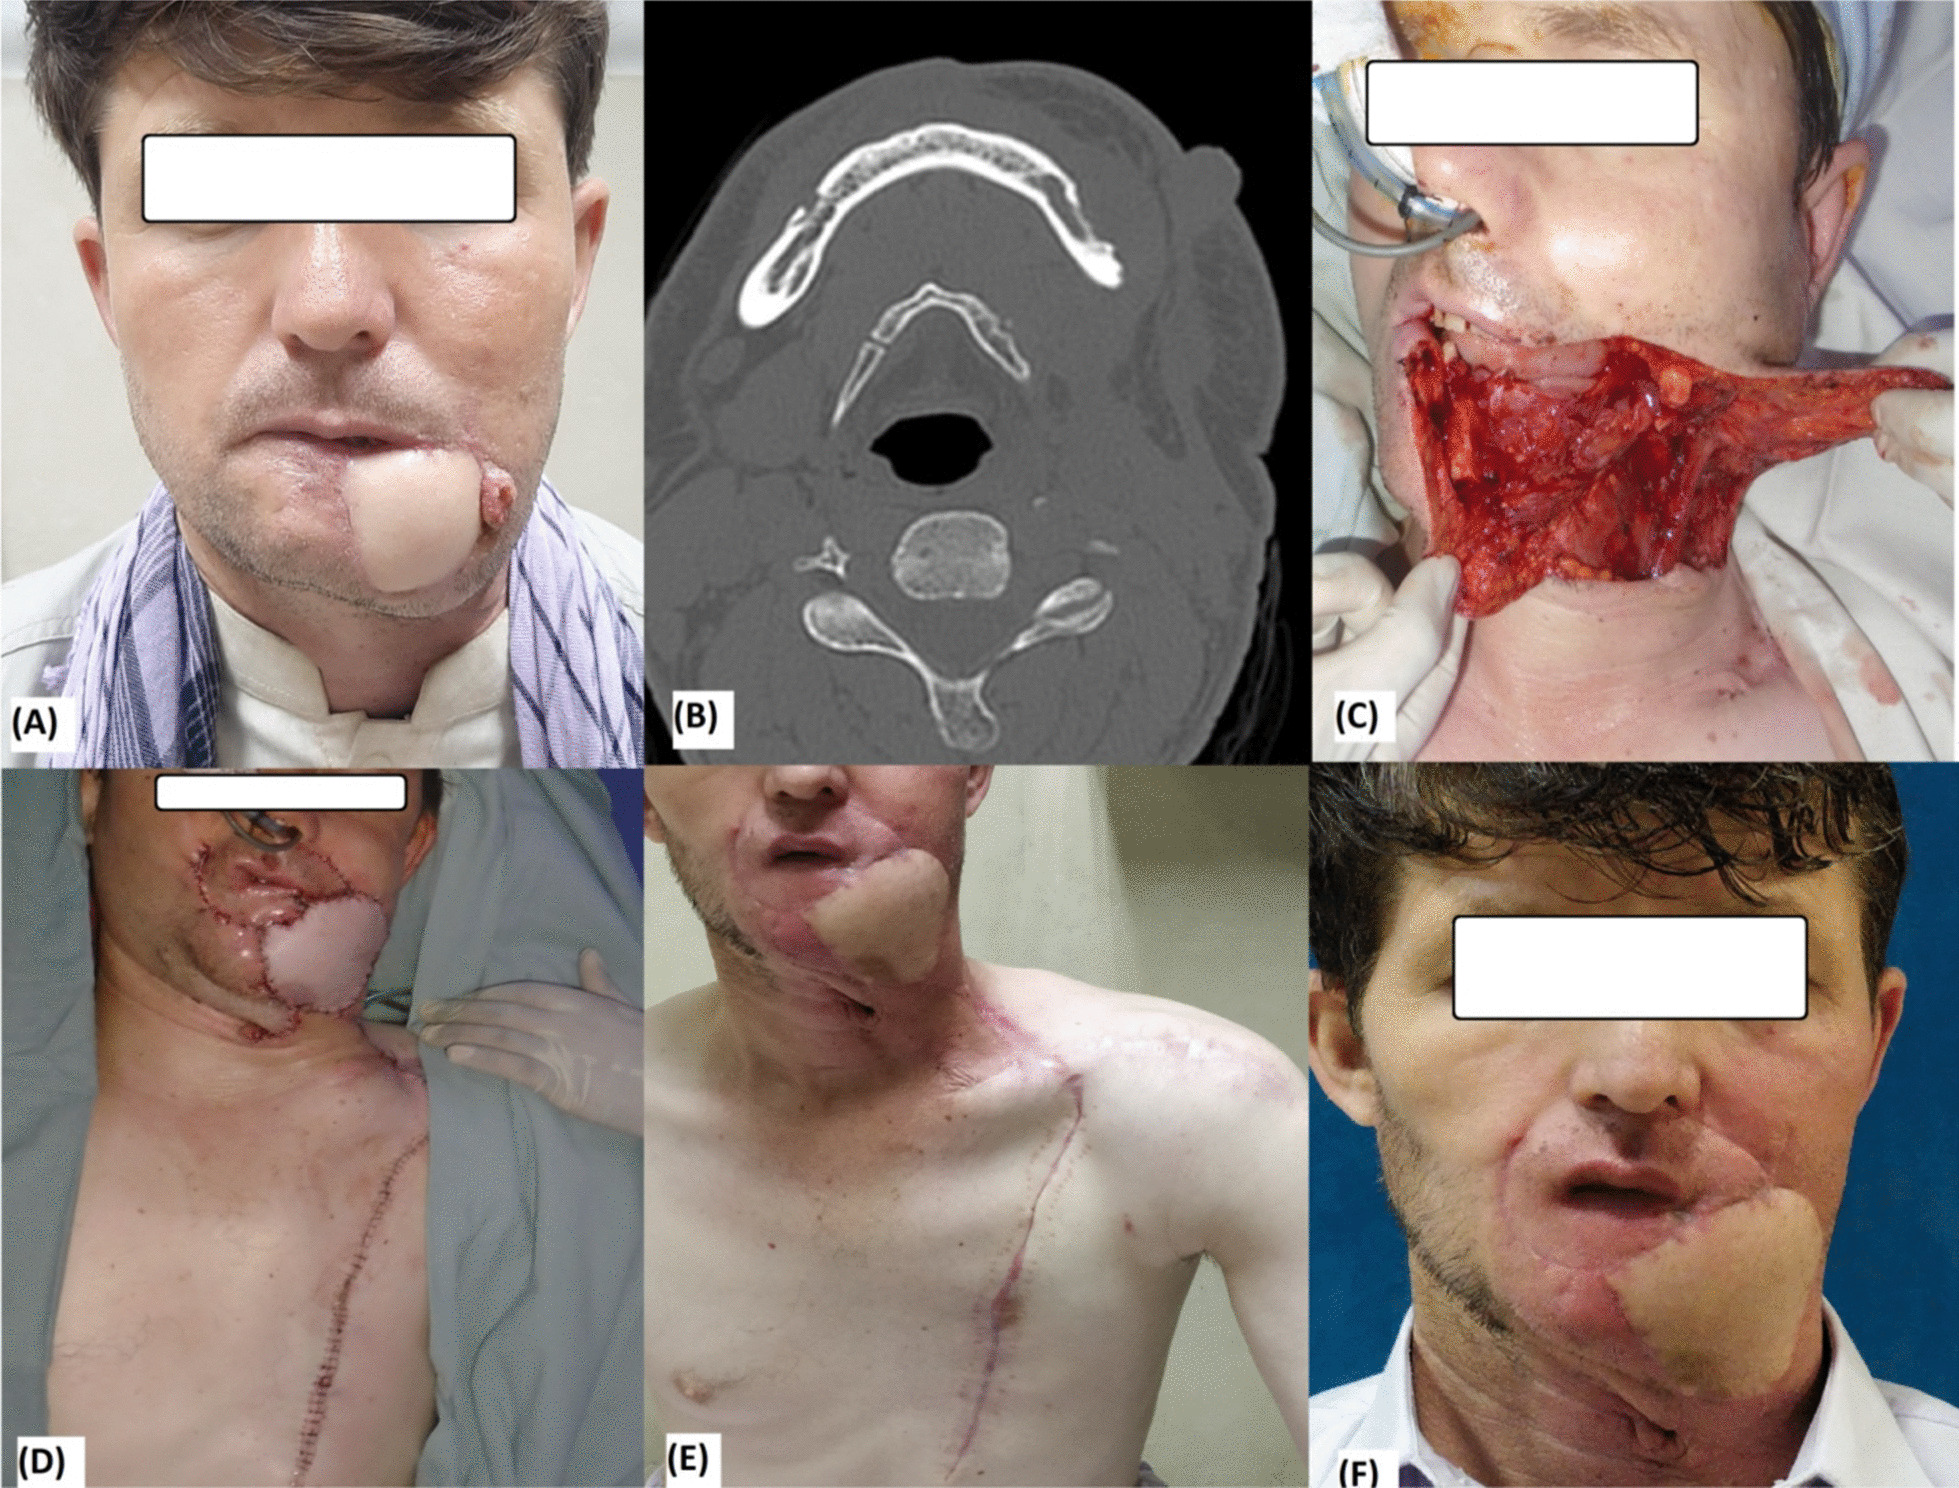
Case 2 a Case 2 Young male with recurrent Squamous cell carcinoma lower lip and mandible. There is a visible supraclavicular flap of previous surgery. b CT scan showing extent of tumor to bone and floor of mouth. c Per-operative view of wide local excision showing soft tissue and boney defect. d Per-operative view showing pectoralis major myocutaneous flap after inset and Karapandzic technique used for lip defect. e Early followup picture. f Frontal view of patient showing nicely healed wounds
